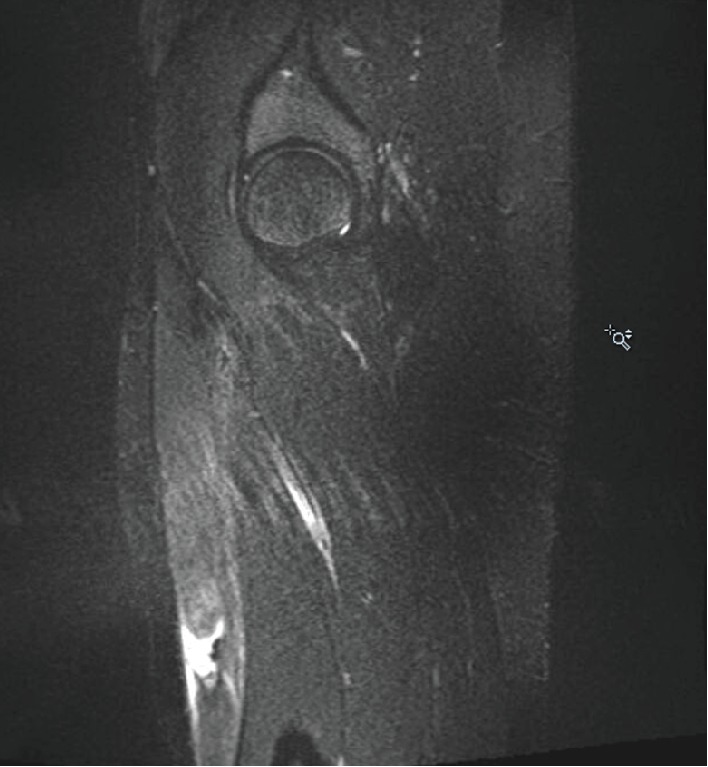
T2 STIR sagittal MRI image of the right femur without contrast demonstrating an acute degloving injury of the right rectus femoris.
